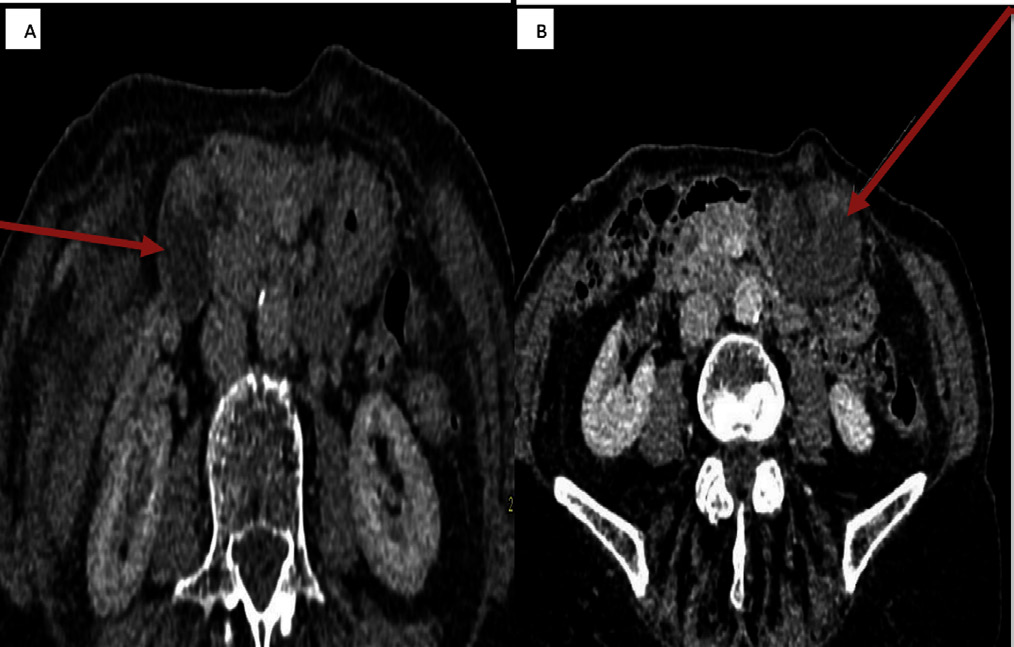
(A) Axial view CT -2018 showing Gall bladder in gall bladder fossa (B) CT -2020 Axial view demonstrating the gallbladder-like structure with evidence of ischaemia on the left side
